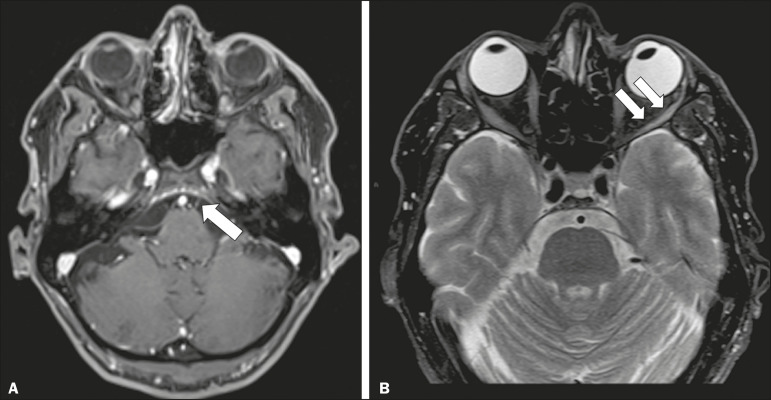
Abducens nerve trauma. A 50-year-old male with convergent strabismus after a motor vehicle accident. A: Axial contrast-enhanced T1-weighted spoiled gradient-recalled MRI sequence showing enhancement of the abducens nerve. B: Axial T2-weighted MRI scan showing a hyperintense signal, consistent with acute denervation, in the left lateral rectus muscle.
